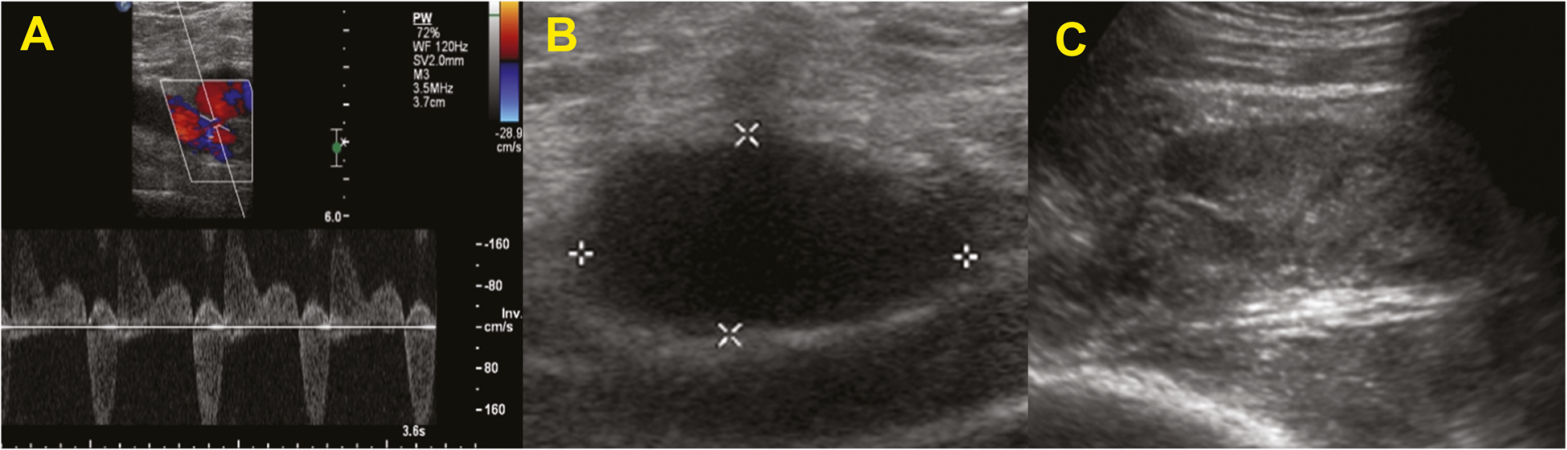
a-c Pseudoaneurysm (PSA) embolized with thrombin. Doppler US view (a) of a common femoral artery PSA with a small neck. Pre-treatment PSA, B-mode examination (b). Thrombosed PSA (c) after treatment with thrombin injection under ultrasound guidance
